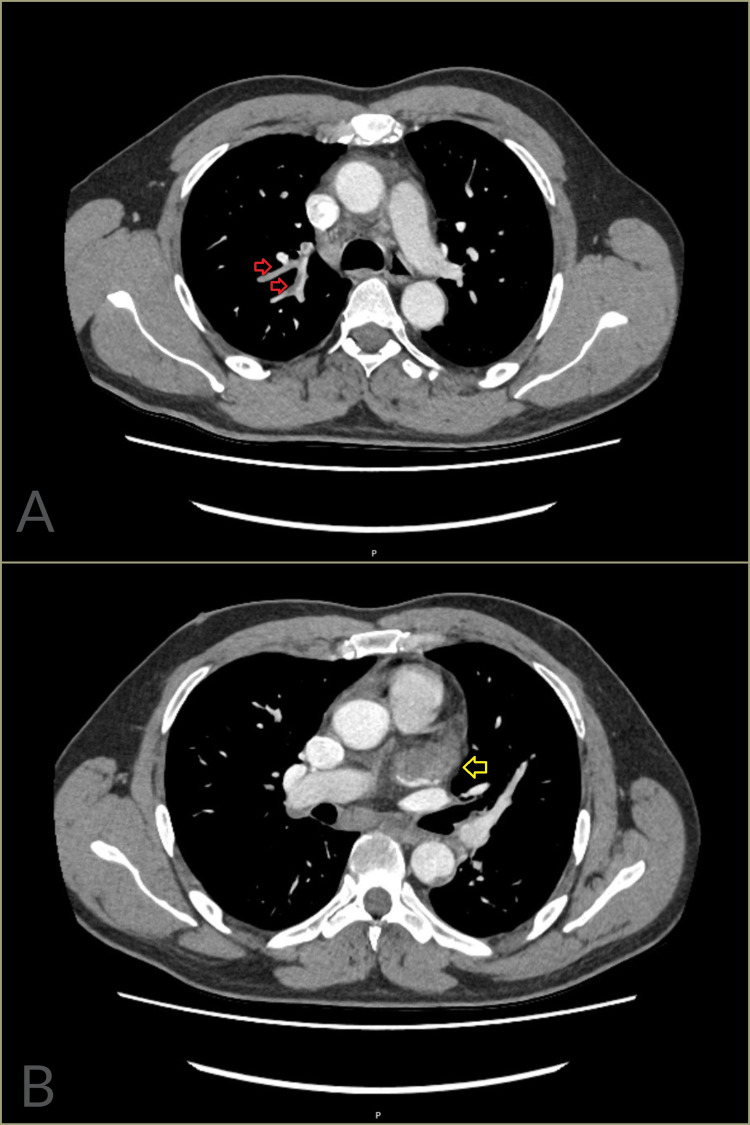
CT of the chest with contrast (Case 1). (A) There is reasonable opacification of the pulmonary arteries demonstrating thrombi in the right lobar branches (red arrows). (B) The left atrial appendage appears enlarged and is largely occluded by thrombus (yellow arrow).
